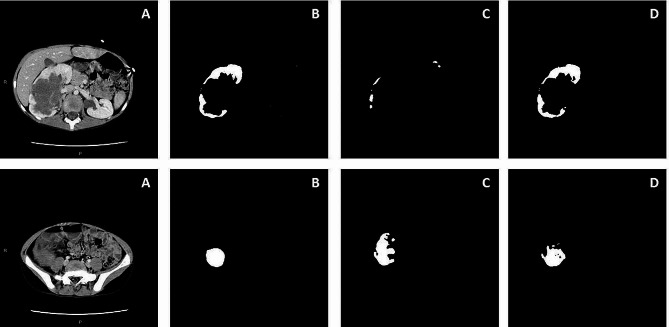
Results of renal (first line) and tumor (second line) segmentations. Column A: CT cross-sections. Column B: manual segmentations performed by a human expert. Column C: automatic segmentations obtained with CNN U-Net. Column D: segmentations obtained with CNN U-Net + OV^2^ASSION training method
